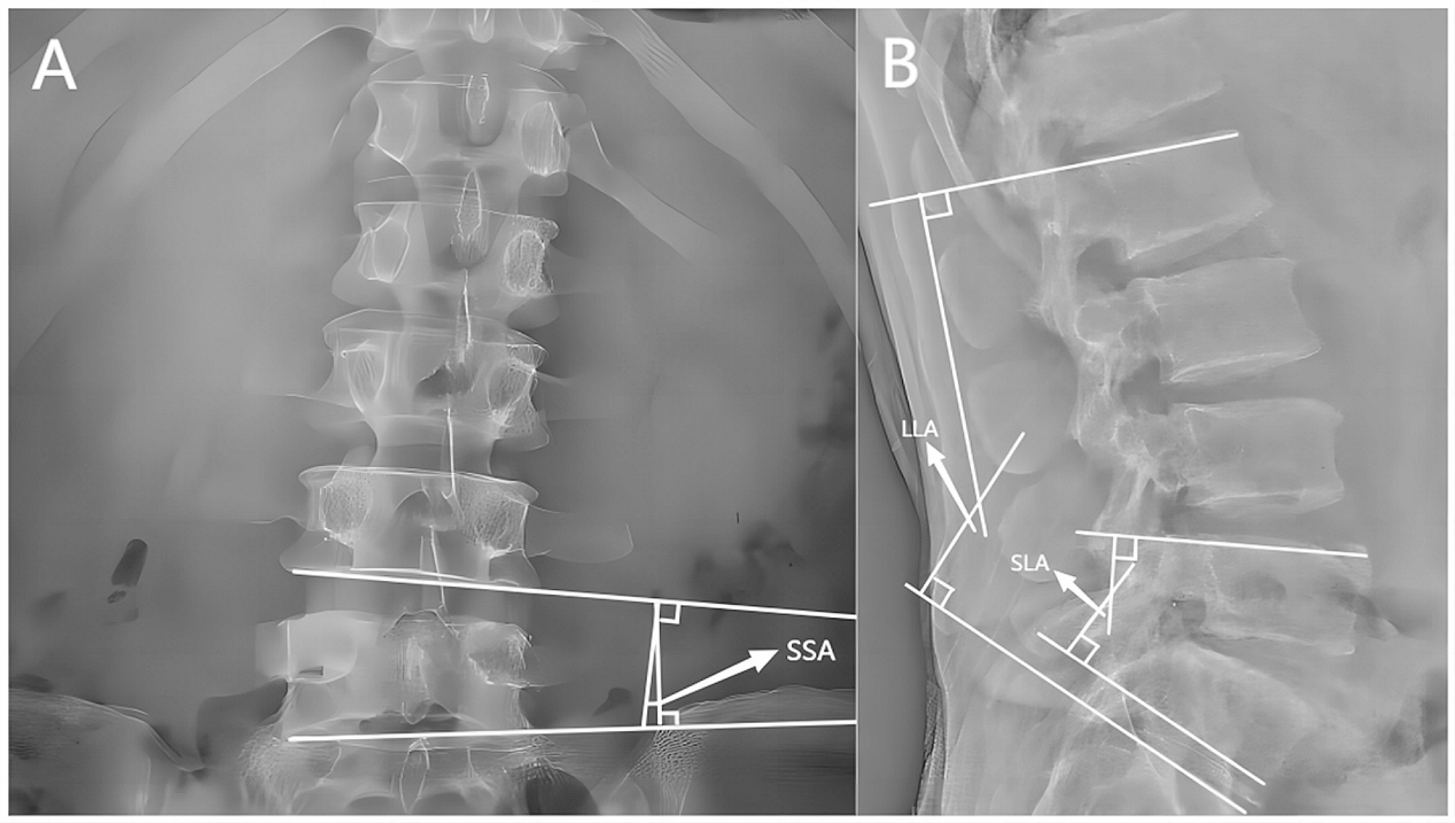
(A) The segmental scoliosis angle (SSA) is the cobb angle between the lower endplate of the superior vertebral body and the inferior endplate of the lower vertebral body on the coronal X-ray film of the surgical segment. (B) The segmental lordotic angle (SLA) is the cobb angle between the superior endplate of the superior vertebral body and the inferior endplate of the lower vertebral body on the sagittal X-ray of the lumbar. The lumbar lordotic angle (LLA) is the cobb angle between the superior endplate of L1 vertebral body and the superior endplate of S1 vertebral body in sagittal X-ray
